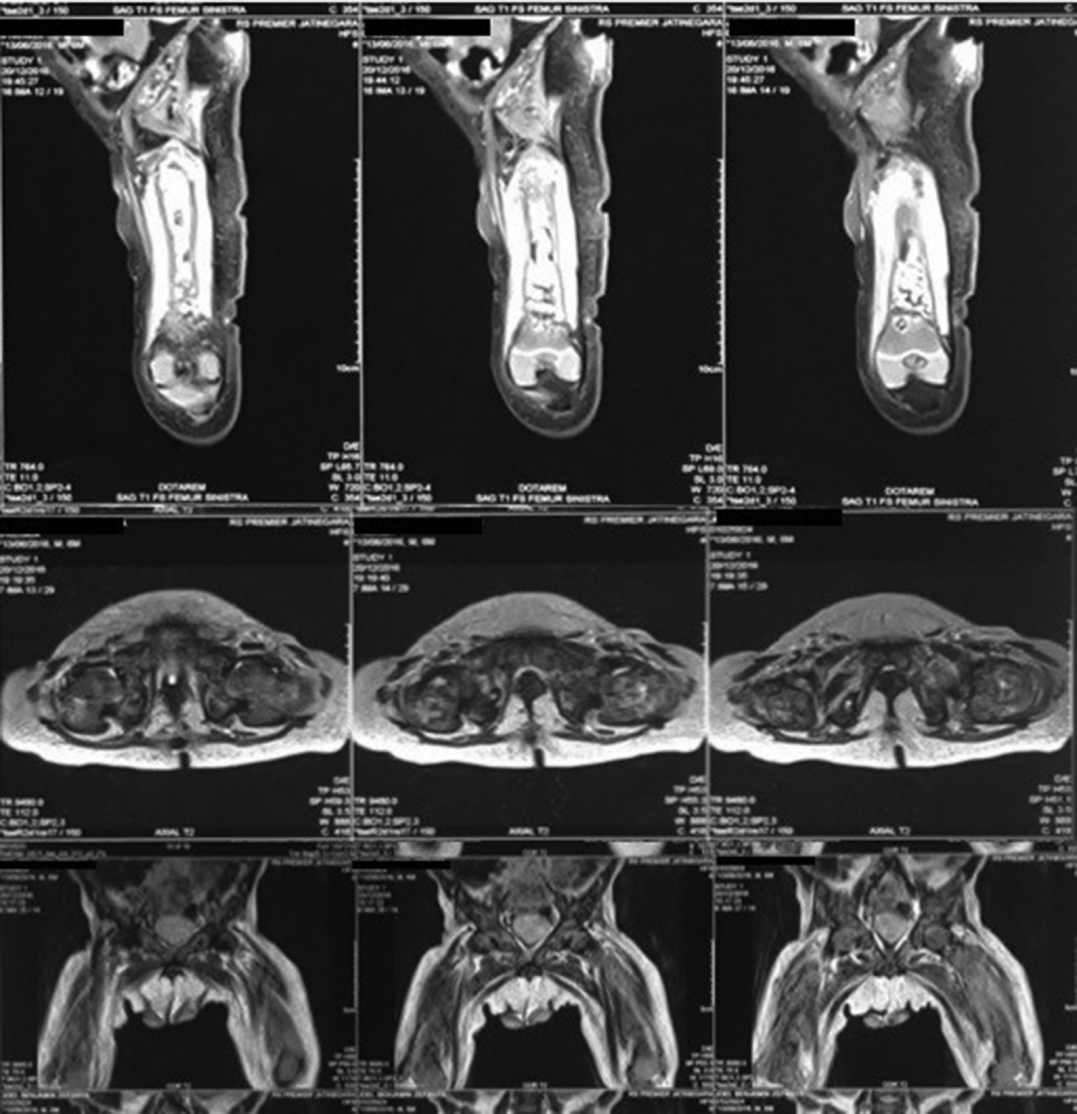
Second MRI was taken to confirm and compare with previous MRI, and the result suggested metastatic neuroblastoma as differential diagnosis.
